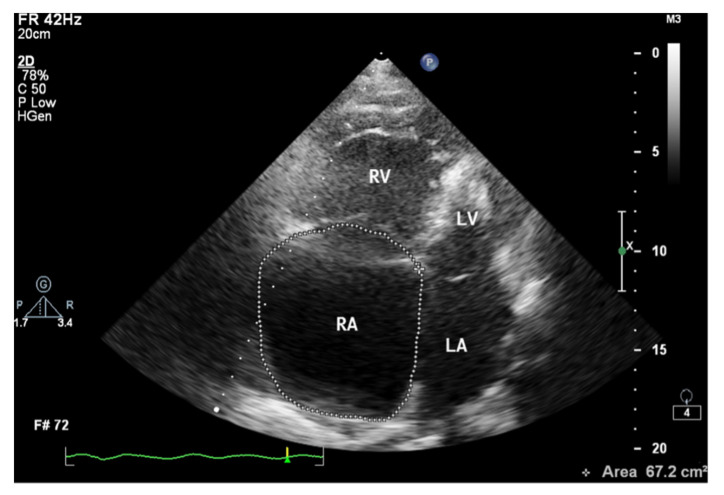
Enlarged right atrium with an area of 67.2 cm^2^. 2D-TTE, 4CH view. 2D-TTE: two-dimensional transthoracic echocardiography; 4CH: four-chamber.
